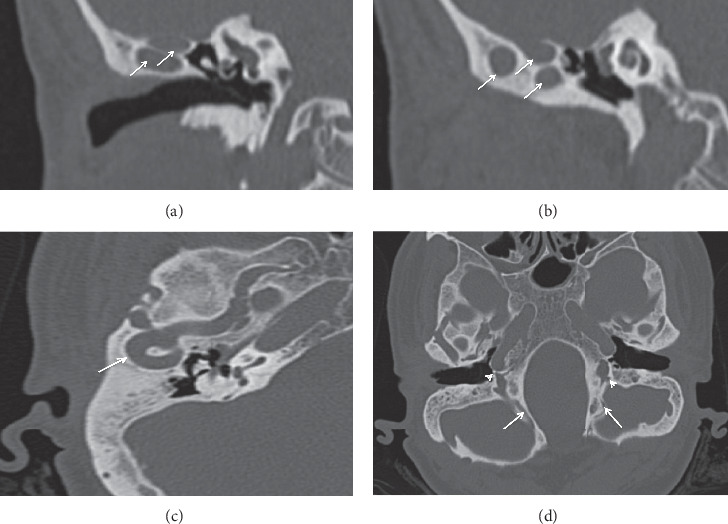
Imaging of the right ear showing enlarged emissary veins (arrows). (a), (b) Coronal HRCT. (c) Axial HRCT. (d) Axial HRCT (mIP), showing small posterior condylar emissary canal on the left (jugular bulb type) and groove on the right (occipital sinus type)—arrows; atresic jugular foramen—arrow heads. HRCT: high-resolution computed tomography; mIP: maximum intensity projection.
